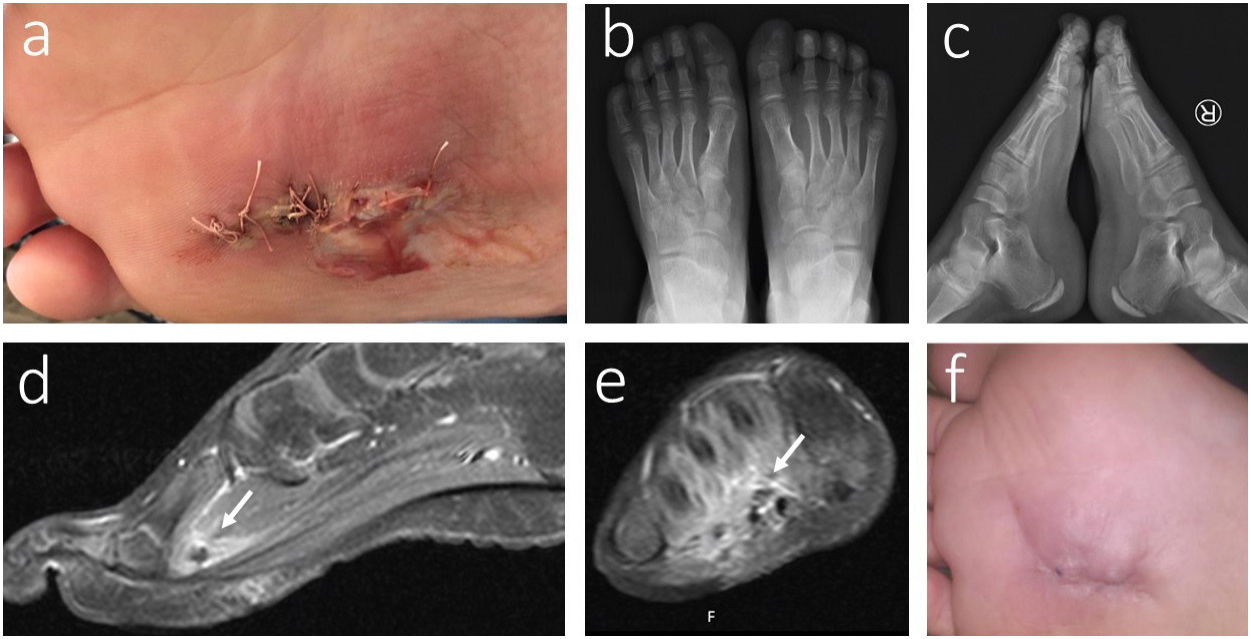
11-year-old male with missed penetrating sponge injury in his right foot. On his admission to our hospital, there was a purulent discharge on his right foot after his first administration to another centre (a); radiographic images showed nothing related with the FB (b,c); ultrasonography revealed 2 FBs in his right foot. After removal of the FBs, a purulent discharge continued up to the follow up period. An MRI was performed for the patient, and the MRI-T2 showed a missed FB between the 2nd and 3rd flexor tendons of the foot (sagittal view (d), axial view (e)); therefore, we understood that the USG did not locate the 3rd FB, and the patient was operated on to remove the 3rd missed sponge FB. The wound healed, and the discharge stopped after the removal of the deep missed FB after the 2nd operation in our hospital.
